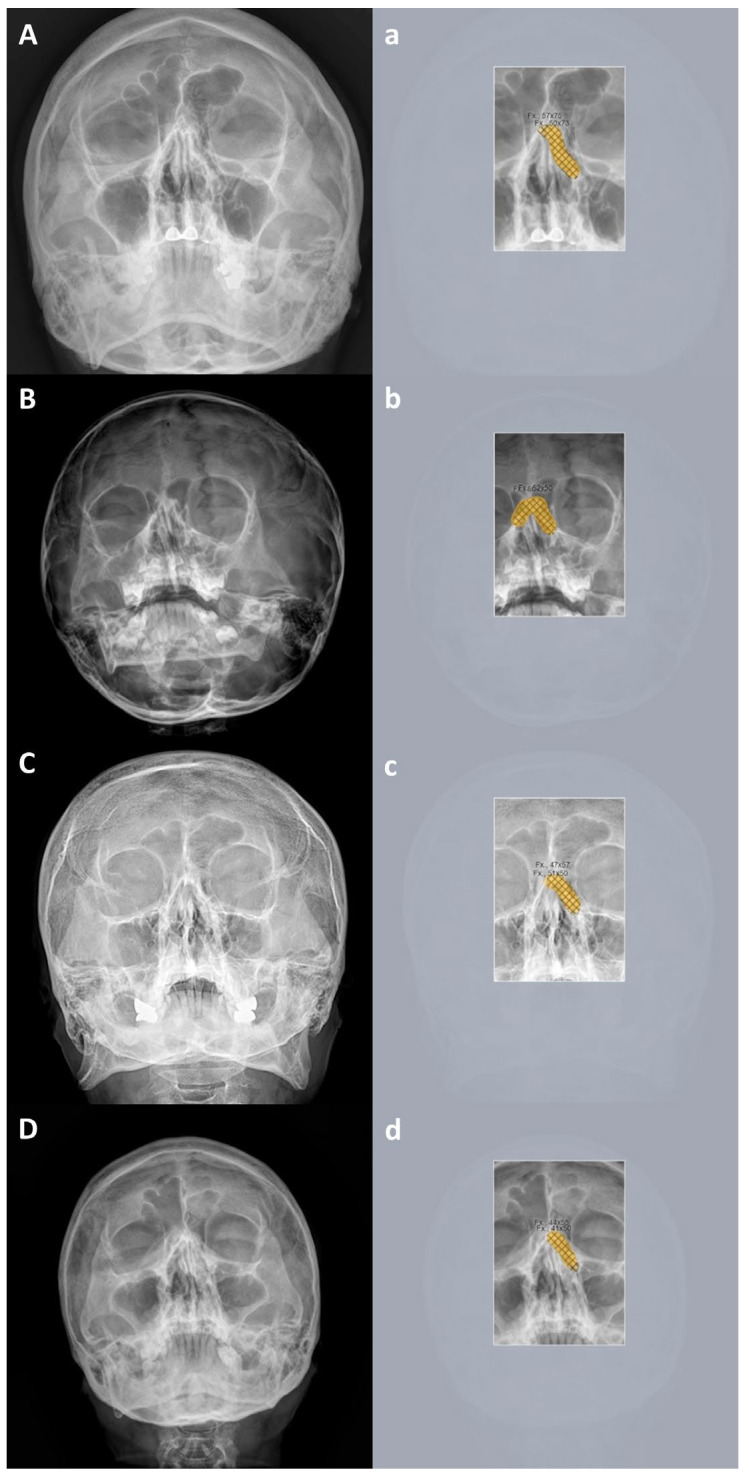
Example images of skull waters’ view radiographs overlaid with segmentation labeling with correct detection. The deep-learning model correctly identified the nasal bone fractures. (Left) Radiographic images of the Skull Waters’ view with the removal of right/left markings from the corners of the X-ray. (Right) The orange color represents the area labeled by the authors using a segmentation method, while the hatched part corresponds to the pixel area predicted as fractures by the deep-learning model. The images (A,a), (B,b) and (C,c) show typical unilateral nasal bone fractures with fracture lines; images (D,d) show comminuted fractures with soft tissue swelling.
